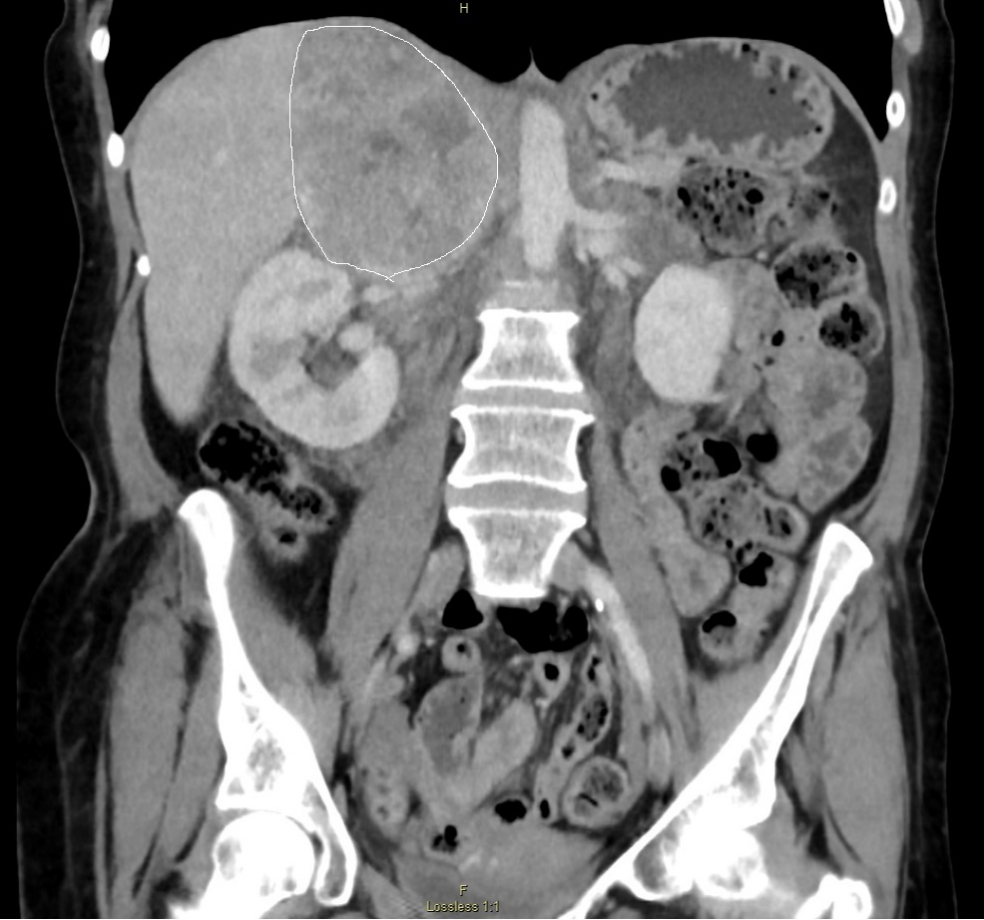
A computed tomography (CT) scan (coronal view) showing a 11.5 cm mass with compression of the vena cava and displacement of the right kidney. The mass is outlined.
